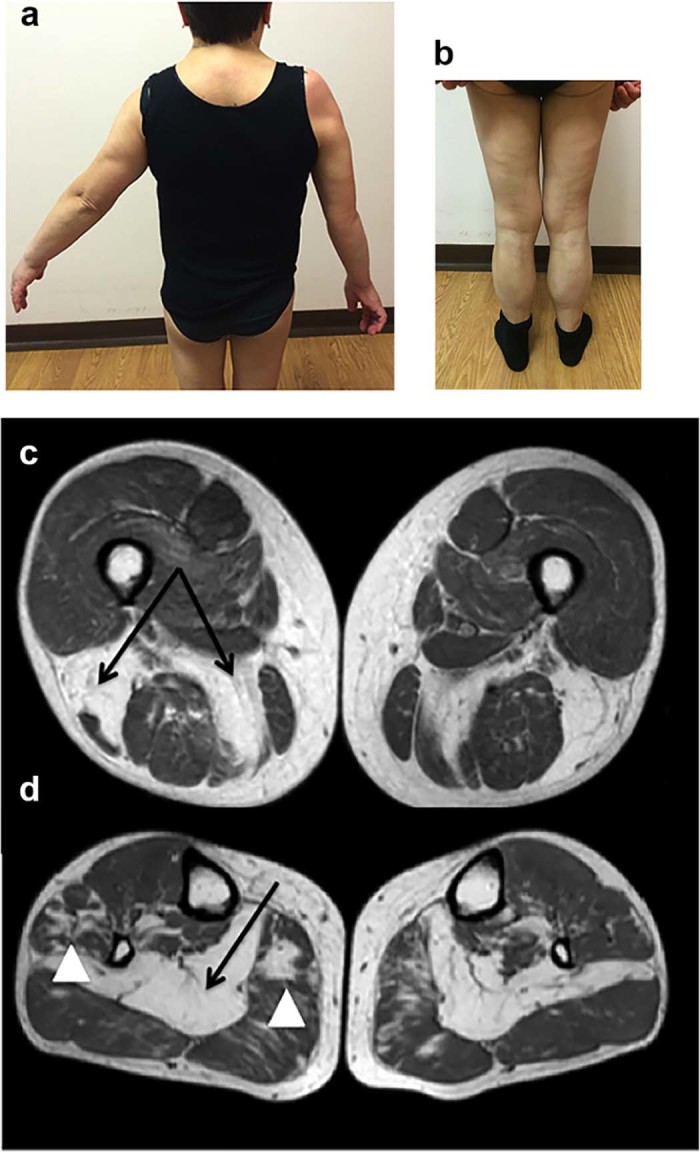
NLSDM patient's clinical and imaging features. (a) Upper limb abduction weakness, predominant on the right side and (b) calves hypertrophy. Thigh (c) and leg (d) muscle MRI: marked T1w changes with almost complete fatty infiltration of biceps femoris and semimembranosus (c) and soleus (d); lower severity involvement has been observed in medial gastrocnemius and peroneal longus (arrowheads) (d).
